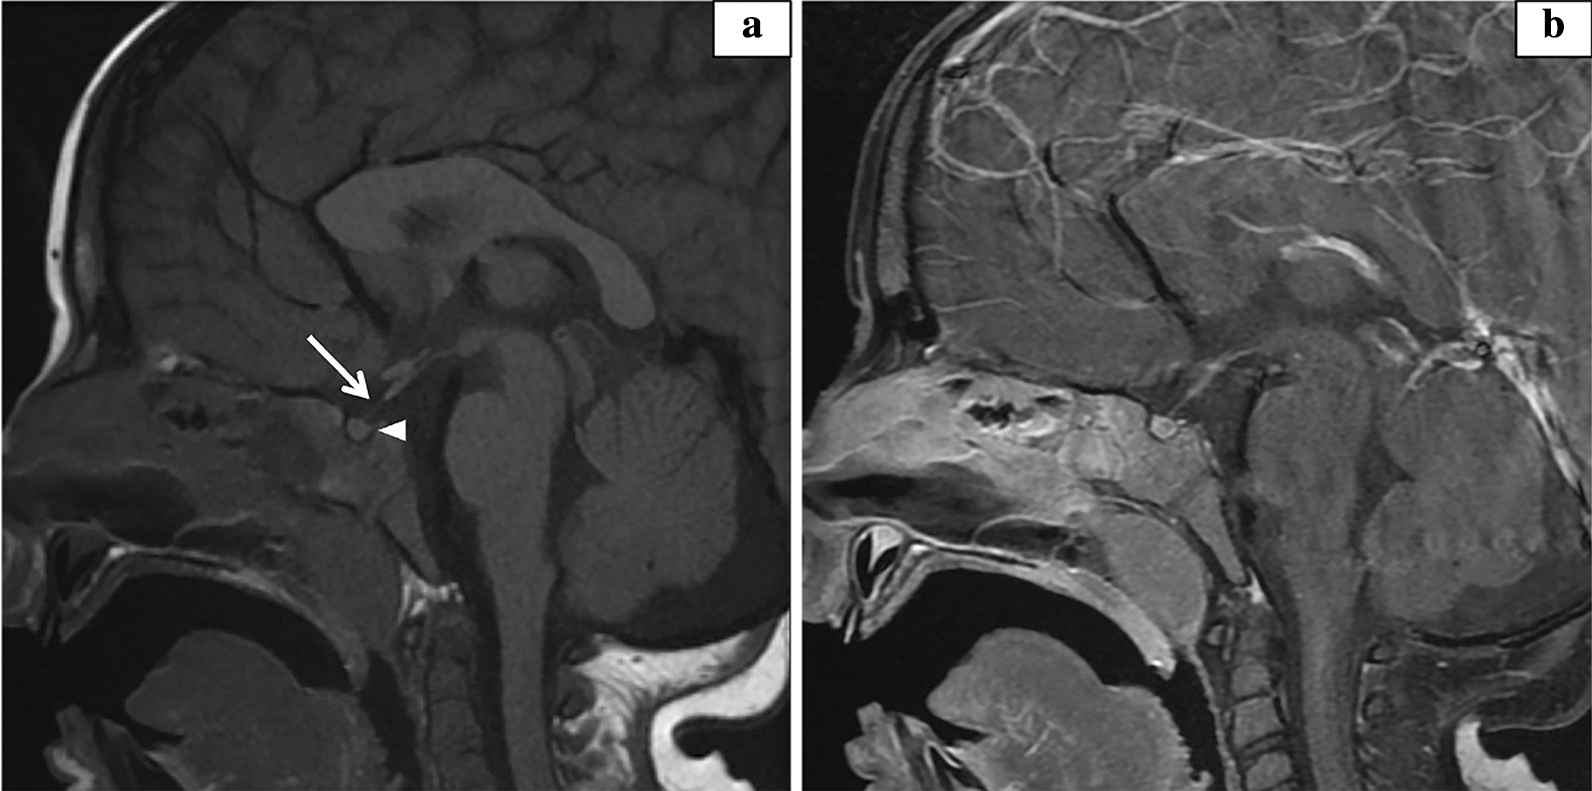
Pituitary magnetic resonance imaging. Sagittal T1 weighted images performed on a 1.5T magnet (GE Healthcare, Chicago, USA). a Pre-contrast T1 weighted images demonstrate nonvisualized/absent neurohypophysis (arrowhead) and abnormal thinning and hypoplasia of the mid to distal infundibular stalk (arrow). b Post-contrast T1 weighted images with fat suppression reveal no pituitary mass
